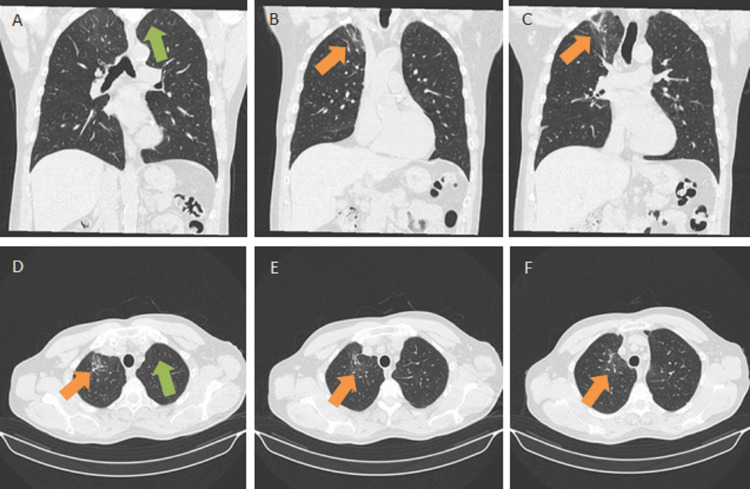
Images of the thoracic computed tomography performed three months after hospitalization (A) Coronal view showing complete resolution of cavitated lesion in the left upper lobe (green arrow). (B and C) Coronal views showing residual fibro-retractable alterations in the right upper lobe (orange arrows). (D) Axial view showing complete resolution of cavitated lesion in the left upper lobe (green arrow). (D, E, and F) Axial views showing residual fibro-retractable alterations in the right upper lobe (orange arrows)
